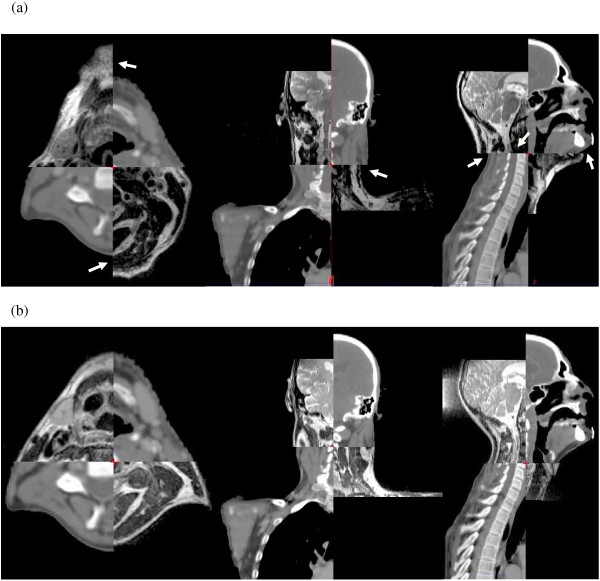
Split view showing the registration in the axial, coronal and sagittal planes of (a) the CT and MRI_D _and (b) the CT and MRI_RT _datasets. Arrows indicate regions where there is a registration mismatch between CT and MRI_D_. Typically, more discrepancies in registration occurred with MRI_D_ than MRI_RT_. MRI_D_: diagnostic MRI scan; MRI_RT_: radiotherapy positioned MRI scan.
